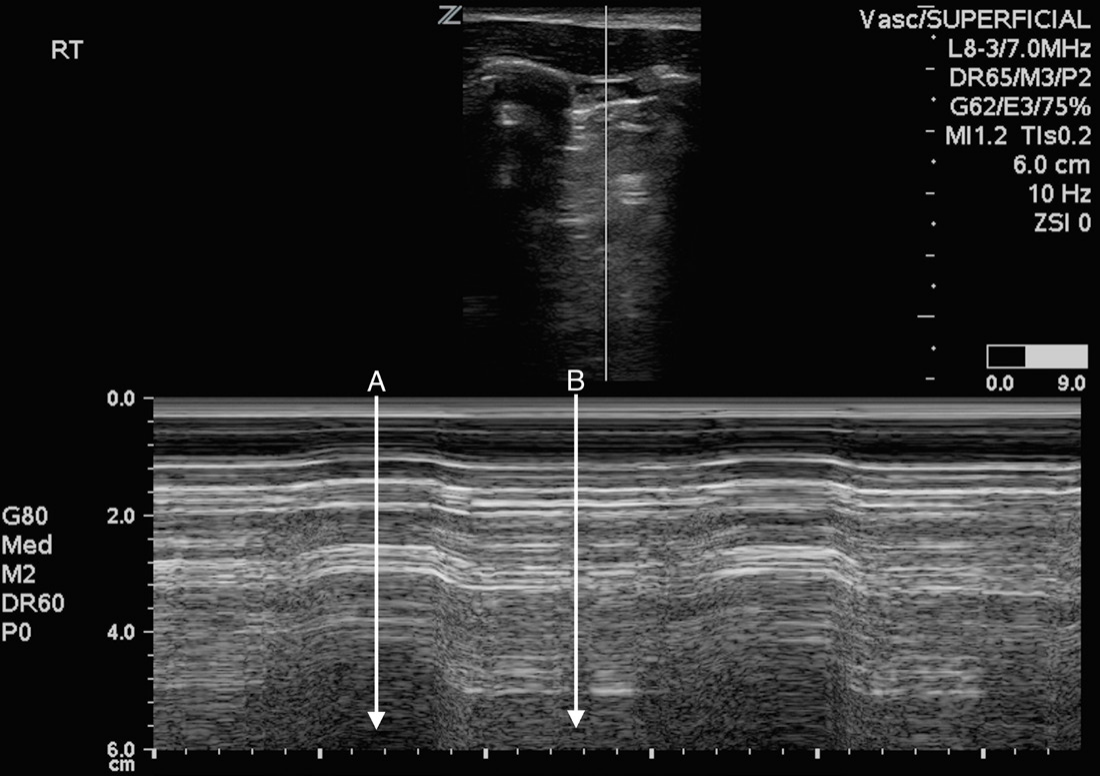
M-mode ultrasound of the right lung, demonstrating bleb point. A. No lung sliding (barcode sign). B. Lung sliding (seashore sign).
